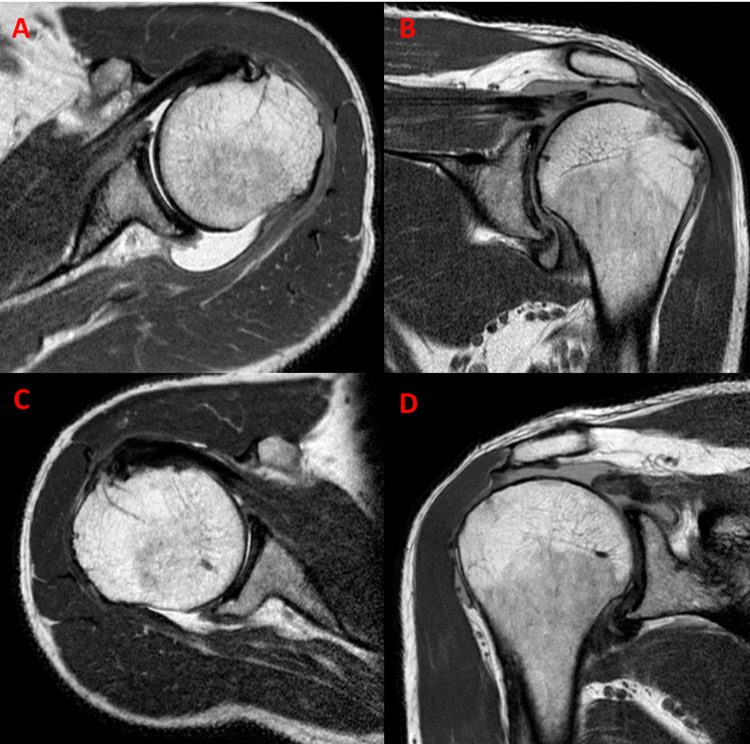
Bilateral shoulder MRI showing irreparable rotator cuff tear on axial and coronal images. Left shoulder (A, B), right shoulder (C, D).
